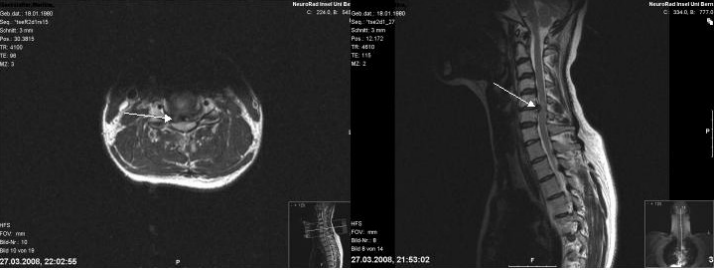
An MRI was obtained in the emergency department for detection of disco-ligamentous injuries. This figure shows T2 weighted transversal and sagittal MRI images. The scan revealed a traumatic extradural rupture of the intervertebral disc between C5 and C6 with ventral myelocompression but without disruption of the dorsal longitudinal ligament
